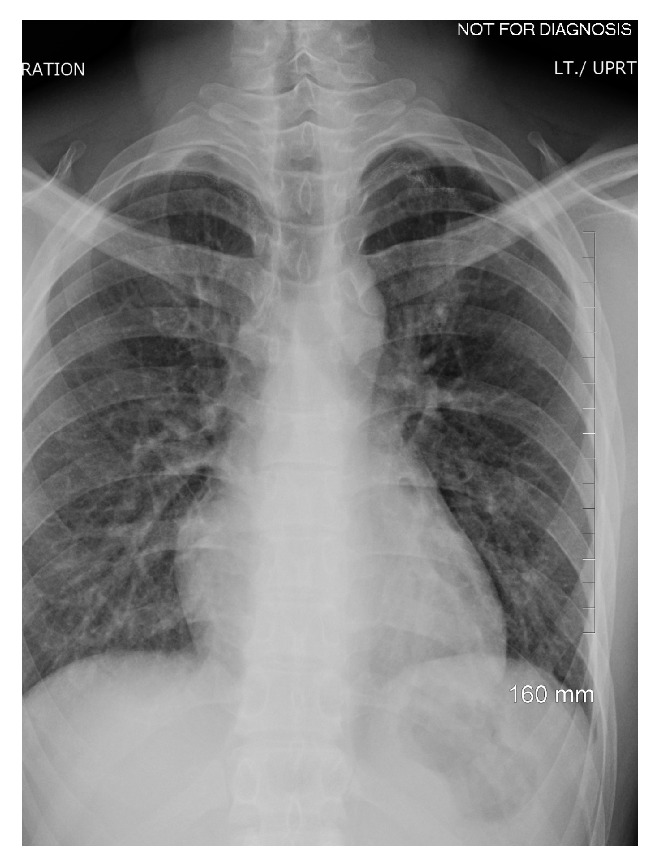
Chest radiograph during outpatient follow-up after the surgery.
